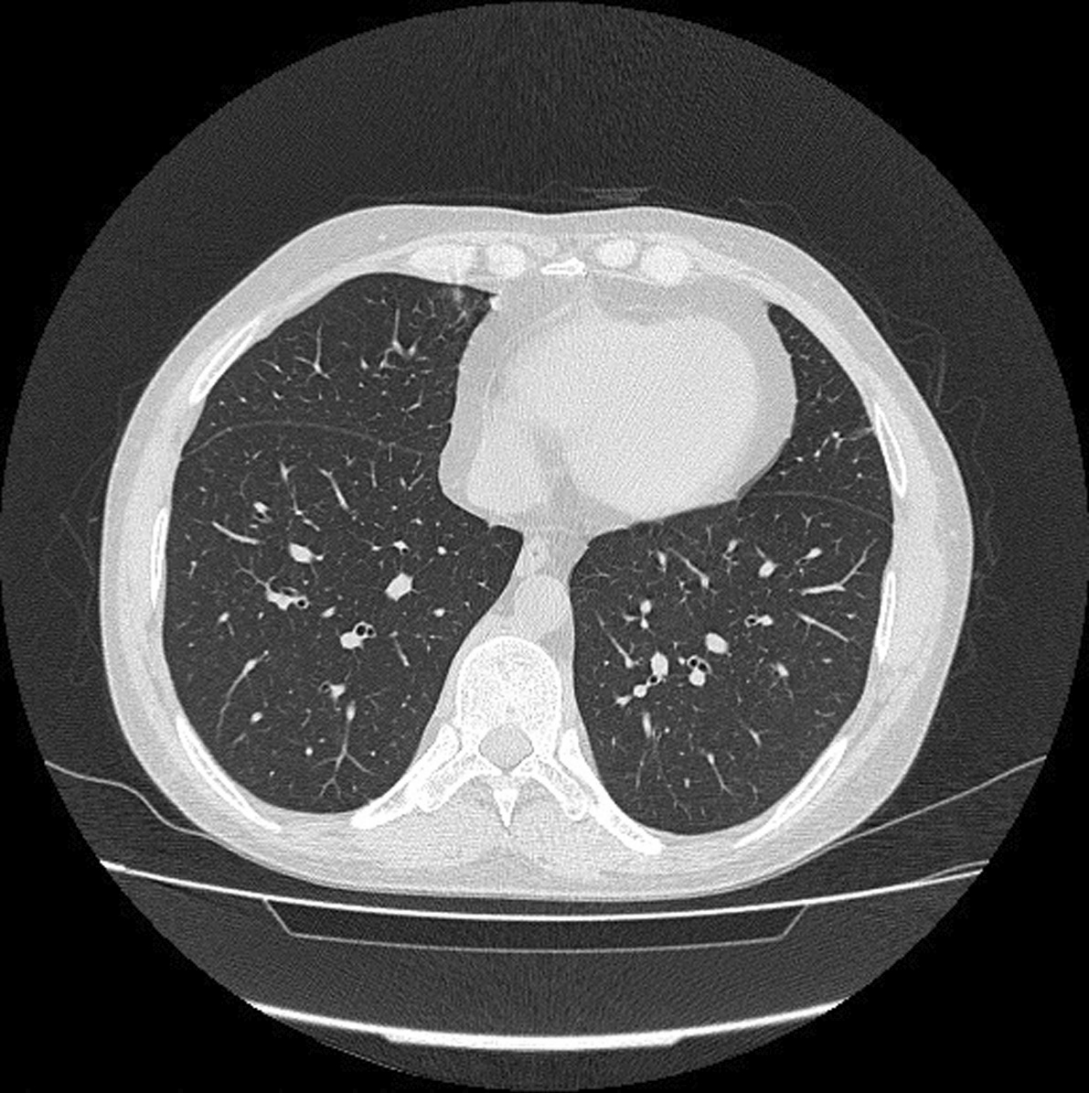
Spinal thoracic computed tomography (CT) scan without intravenous (IV) contrast. The CT scan shows mild grand glass opacity (GGO) and micronodules on the posterior side of both lungs.
